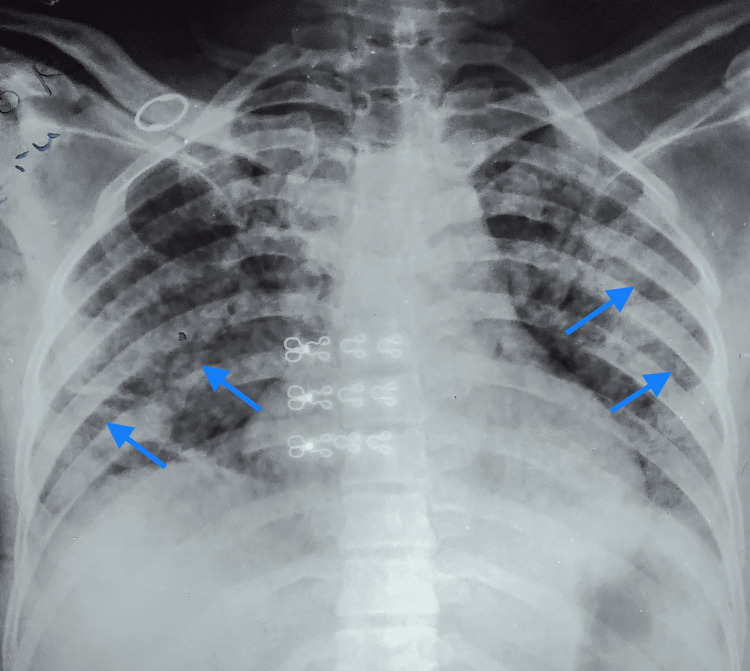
Chest x-ray on admission, showing bilateral non-homogenous opacities (blue arrows) without cardiomegaly consistent with ARDS. ARDS: acute respiratory distress syndrome.
